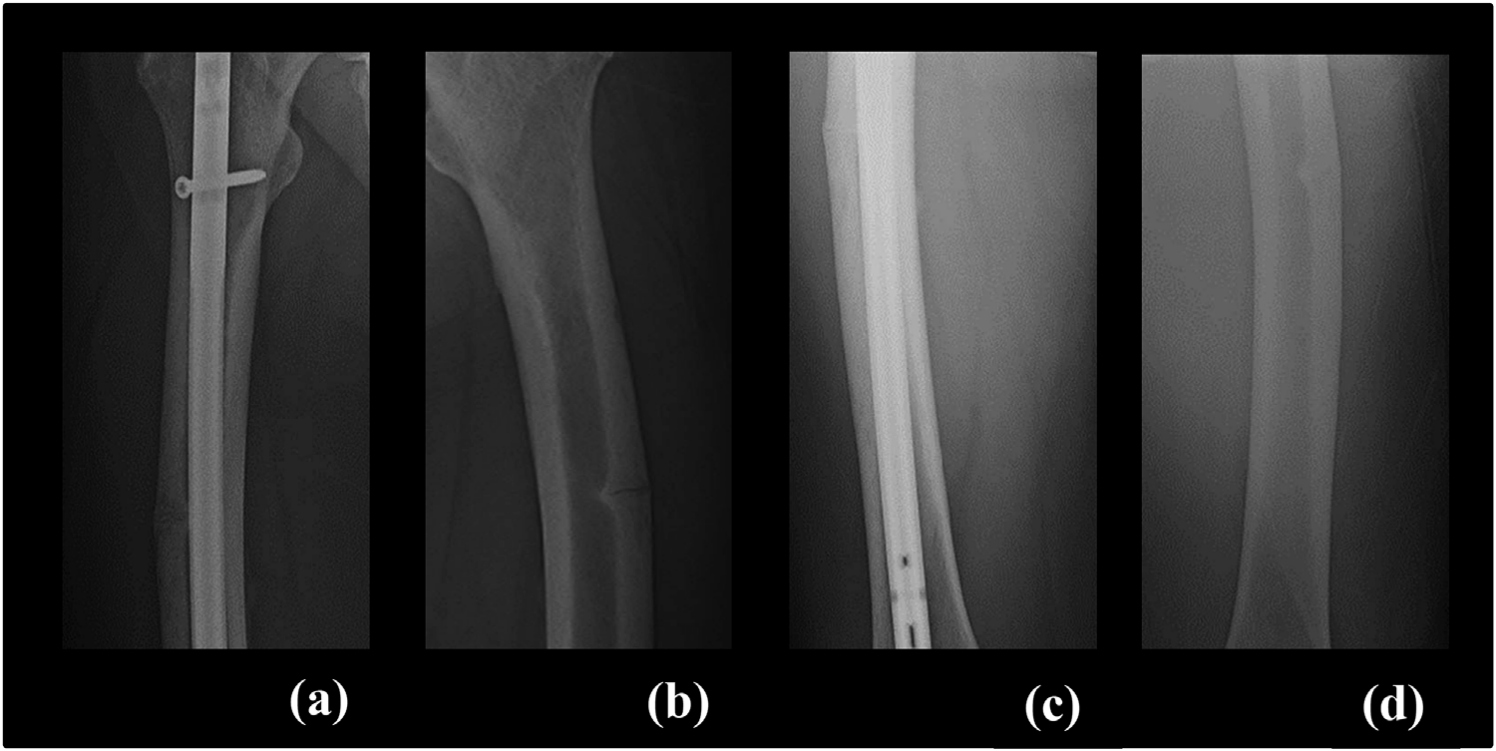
Case 2: Radiograph series of left and right femoral fractures. (*A*) Right femur 9 years after fracture; before asfotase alfa treatment. (*B*) Left femur; before asfotase alfa treatment. (*C*) Right and (*D*) left femur 16 months on medication.
